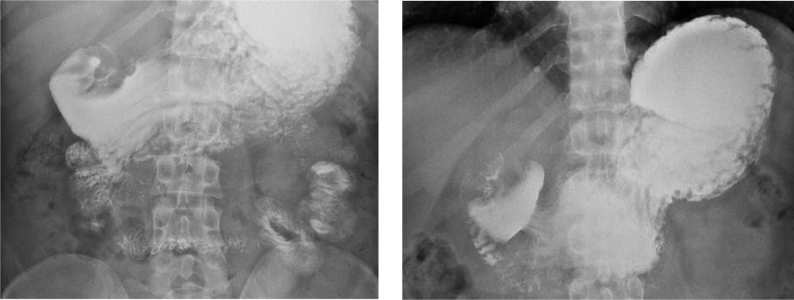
Abdominal X-rays after barium ingestion, showing duodenal obstruction (left and right).
